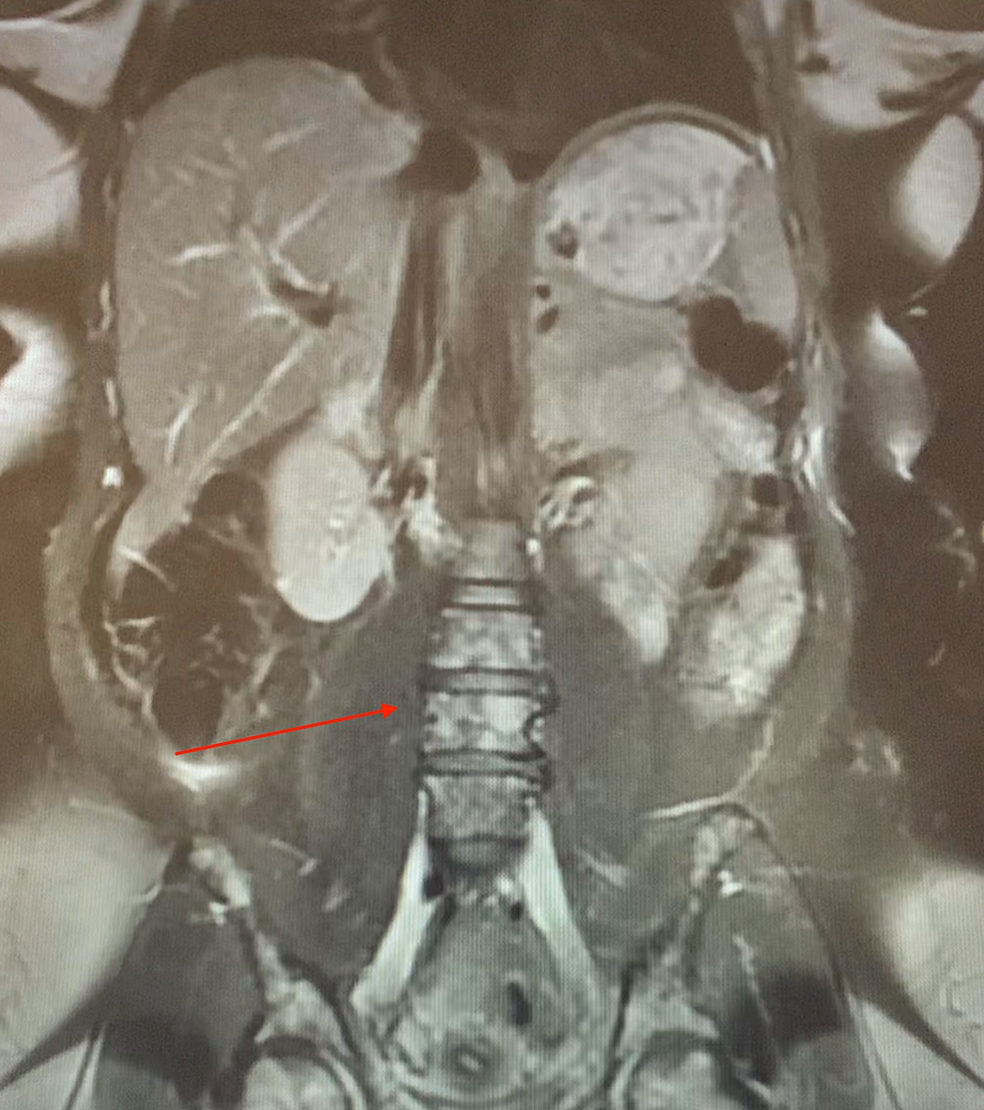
MRI of the lumbar spine without contrast showing diffuse lytic lesions (red arrow).
